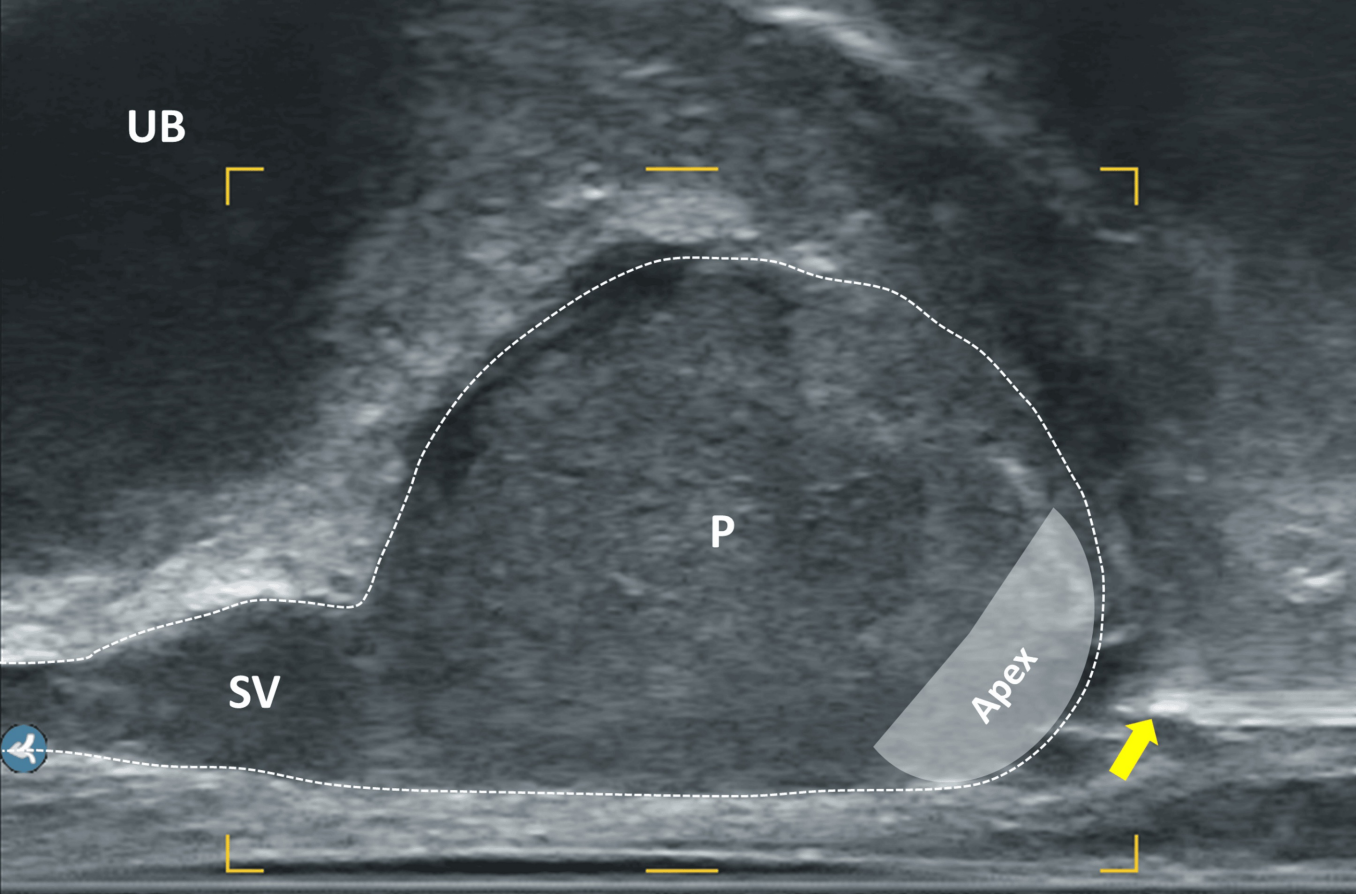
Sagittal section of the prostate, ultrasound probe medially UB: urinary bladder; P: prostate; SV: seminal vesicle; yellow arrow: needle tip in the area of interest in the preapical triangle with local anaesthetic; grey area: apex Image Credit: Toni Franz, corresponding author
